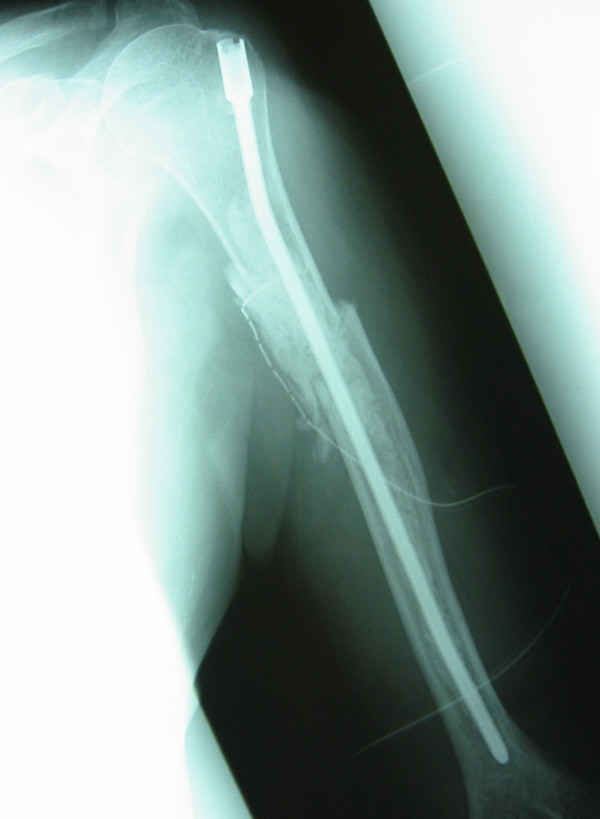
Radiograph of Alta humeral nail. Post operative radiograph of the Alta humeral nail inserted for treatment of a pathological fracture of the humeral diaphysis. Cement can be seen in situ around the nail which is injected via the proximal entry hole prior to insertion of the nail.
